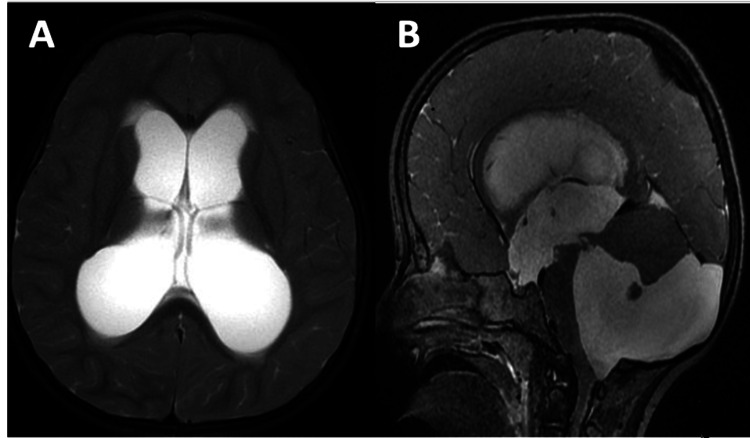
Preoperative MRI brain. (A) Axial cut image shows acute hydrocephalus with transependymal fluid permeation; (B) sagittal image shows large posterior fossa cyst communicating with the fourth ventricle severe compression of the brain stem, cerebellum, and vermis, as well as a dilated supratentorial ventricular system.
